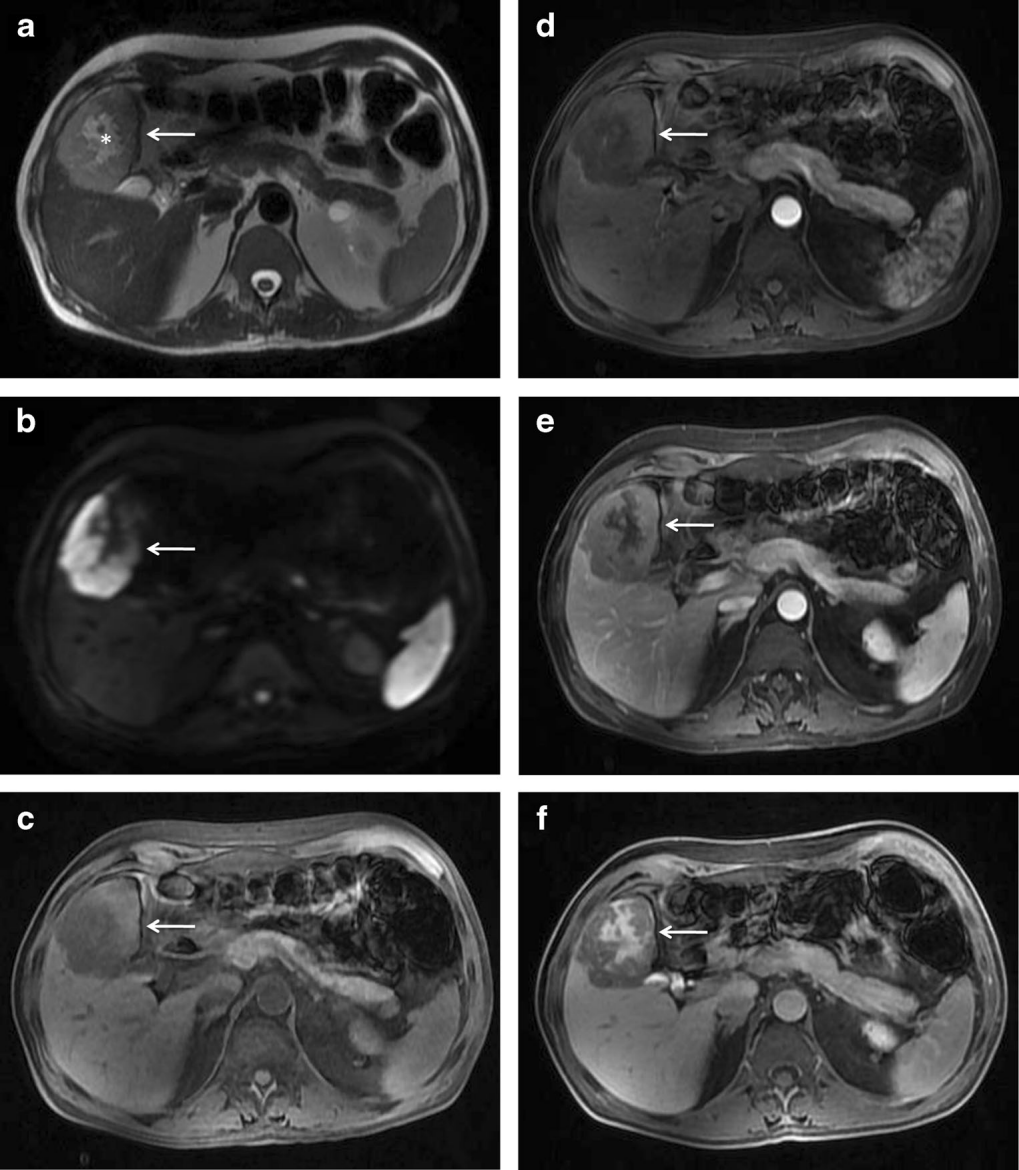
Diffuse large B-cell lymphoma with secondary liver involvement in a 57-year-old man. (a) Axial T2-weighted MR image demonstrating a well-defined, lobulated, mildly hyperintense lesion (arrow) with a more hyperintense central “scar.” It shows peripheral diffusion restriction (arrow in b). The lesion is hypointense on T1-weighted image (arrow in c). On contrast-enhanced scan the lesion shows mild progressive enhancement in the arterial and venous phase images (arrows in d and e), which is less than the surrounding liver parenchyma while the scar remains predominantly non-enhancing. On the 1-hr delayed hepatobiliary phase, the lesion remains hypointense to the surrounding liver parenchyma while there is retention of contrast within the scarThe other pattern is that of enhancement of the rim of the lesion with a central non-enhancing area giving a target-like appearance to the lesion [29, 34]. Due to the well-defined, lobulated outline of these lesions and the predominantly peripheral enhancement, which persists even on the equilibrium phase images, they can be misdiagnosed as intrahepatic cholangiocarcinoma on imaging (Fig. 6). But, unlike a cholangiocarcinoma, capsular retraction and mass effect in the form of biliary dilatation and vascular displacement is typically absent in hepatic lymphoma. Also, CA-19.9 levels are often significantly increased in cholangiocarcinoma.
